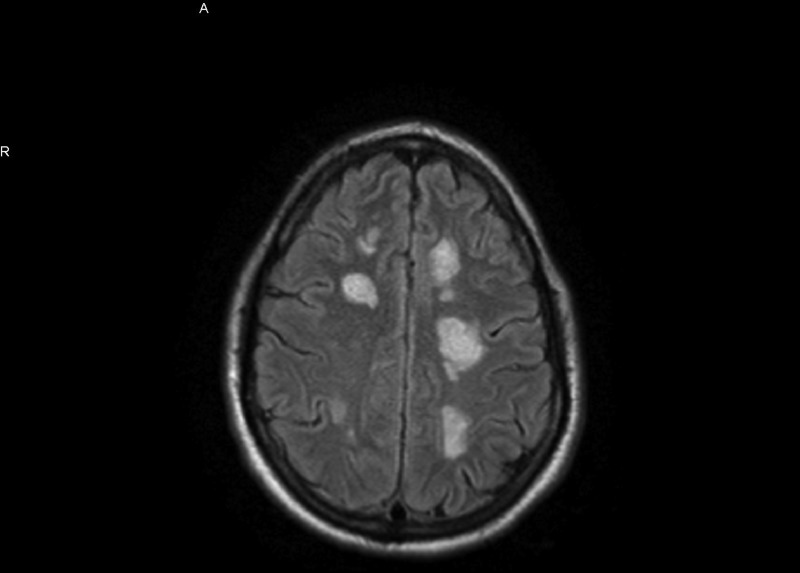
MRI with contrast shows ring-enhancing multiple bilateral lesions. Bilateral white lesions seen in MRI
